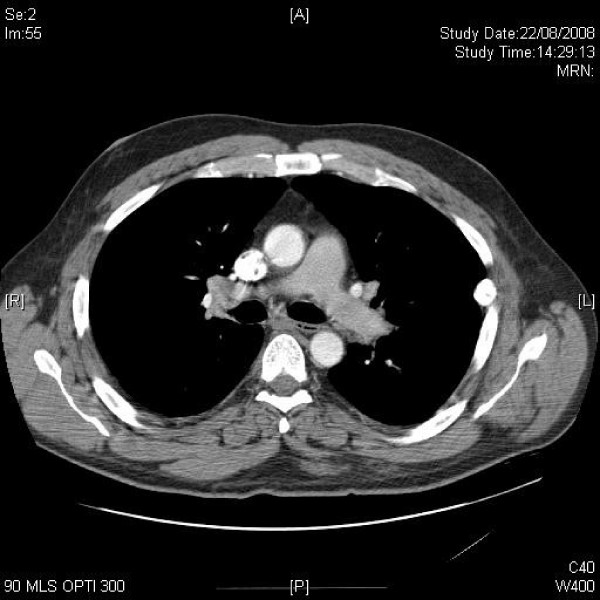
Axial CT of lung hila shows hilar lymphadenopathy.
